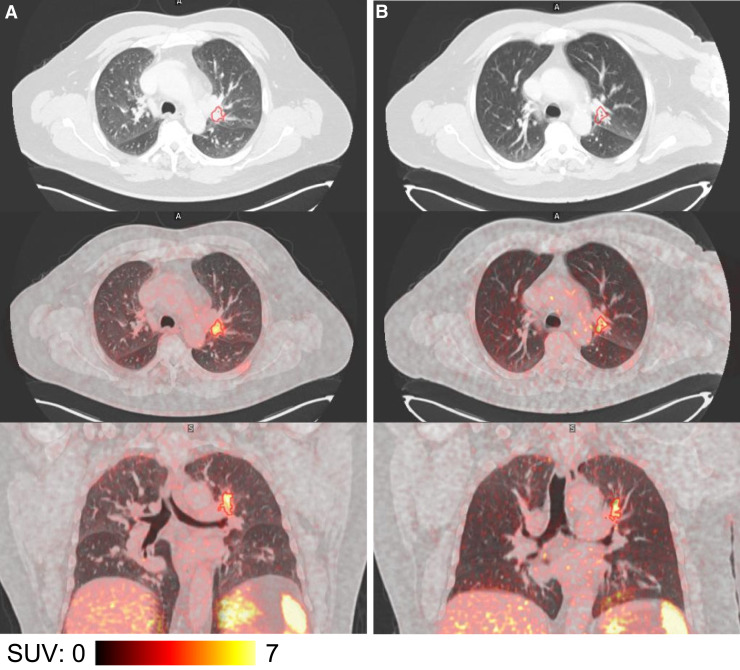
[^68^Ga]Ga-SSO120 PET/CT in patient with SCLC patient who was scanned twice 1.5 mo between and after 4 (A) and 6 cycles (B) of carboplatin plus etoposide. Panels shows transversal CT (upper row), fused transversal [^68^Ga]Ga-SSO120 PET/CT (middle row), and fused coronal [^68^Ga]Ga-SSO120 PET/CT images (lower row). Primary tumor is segmented by SUV of greater than 4 (red line). On CT, size of primary tumor was reduced from 23 × 16 mm to 14 × 11 mm. [^68^Ga]Ga-SSO120 PET showed no clear difference in visual appearance or PET quantification of primary tumor (SUV_max_, 8.8 vs. 9.6; SUV_mean_, 4.9 vs. 5.1).
